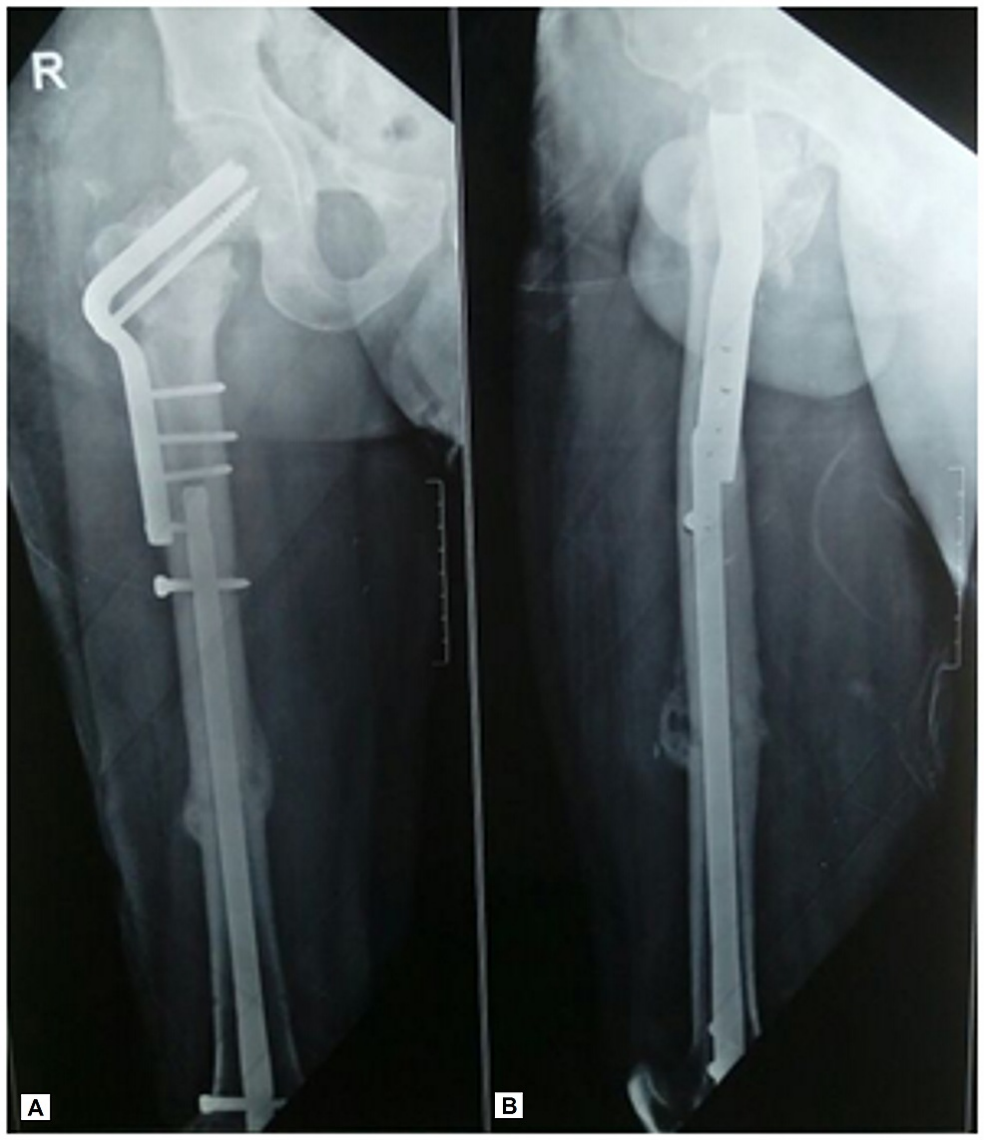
(A, B) Radiographs taken three months postoperatively showing union at the femoral shaft
